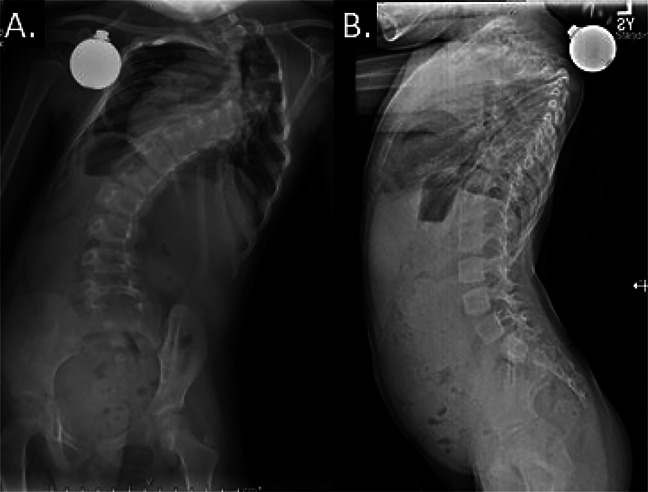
Preoperative radiographic images demonstrating early-onset scoliosis. A, PA view demonstrating the coronal scoliotic curve. B, Lateral view demonstrating the sagittal curve.
